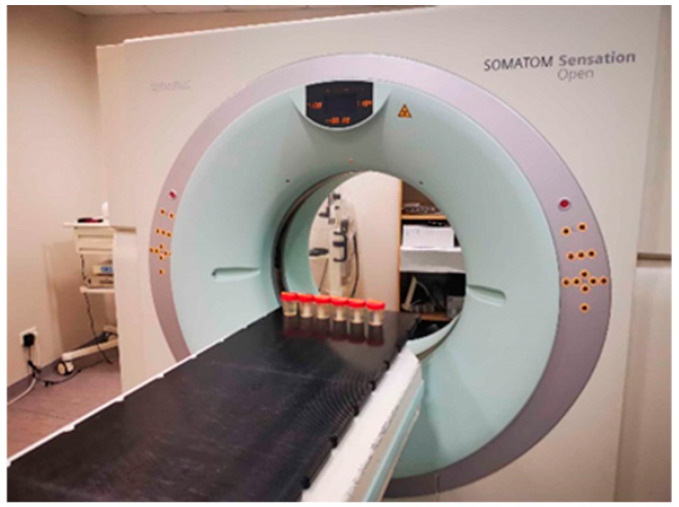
X-ray CT scanning set up for irradiated samples.
